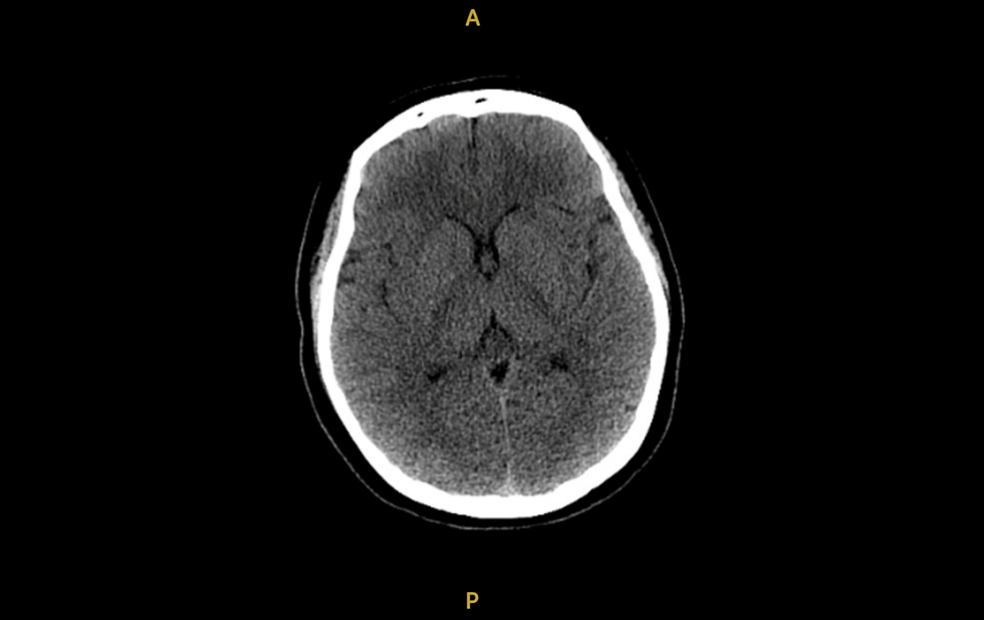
Non-contrast computed tomography (CT) brain of Patient X.
